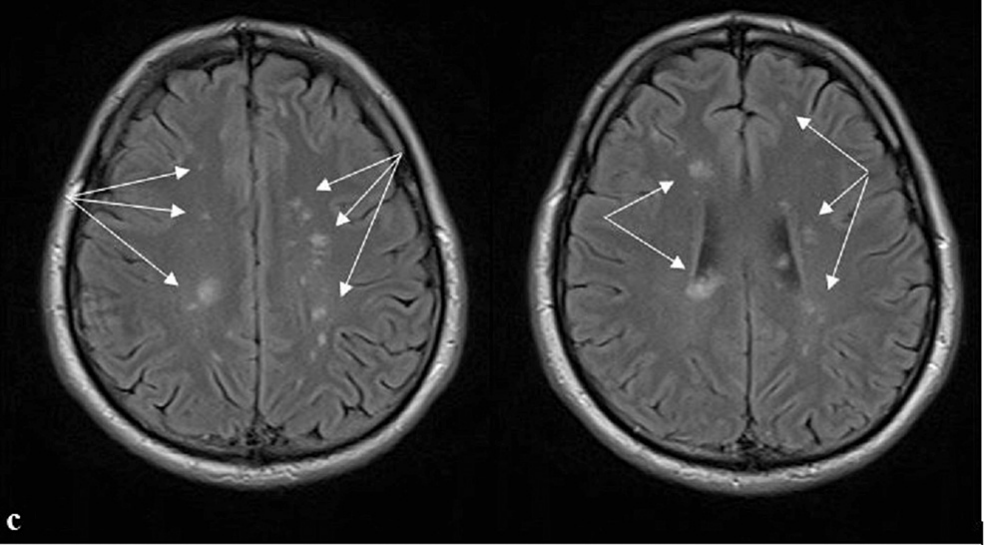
Axial FLAIR MRI of the brain showing multiple hyperintense lesions in the periventricular and juxtacortical white matter FLAIR MRI: fluid-attenuated inversion recovery magnetic resonance imaging
